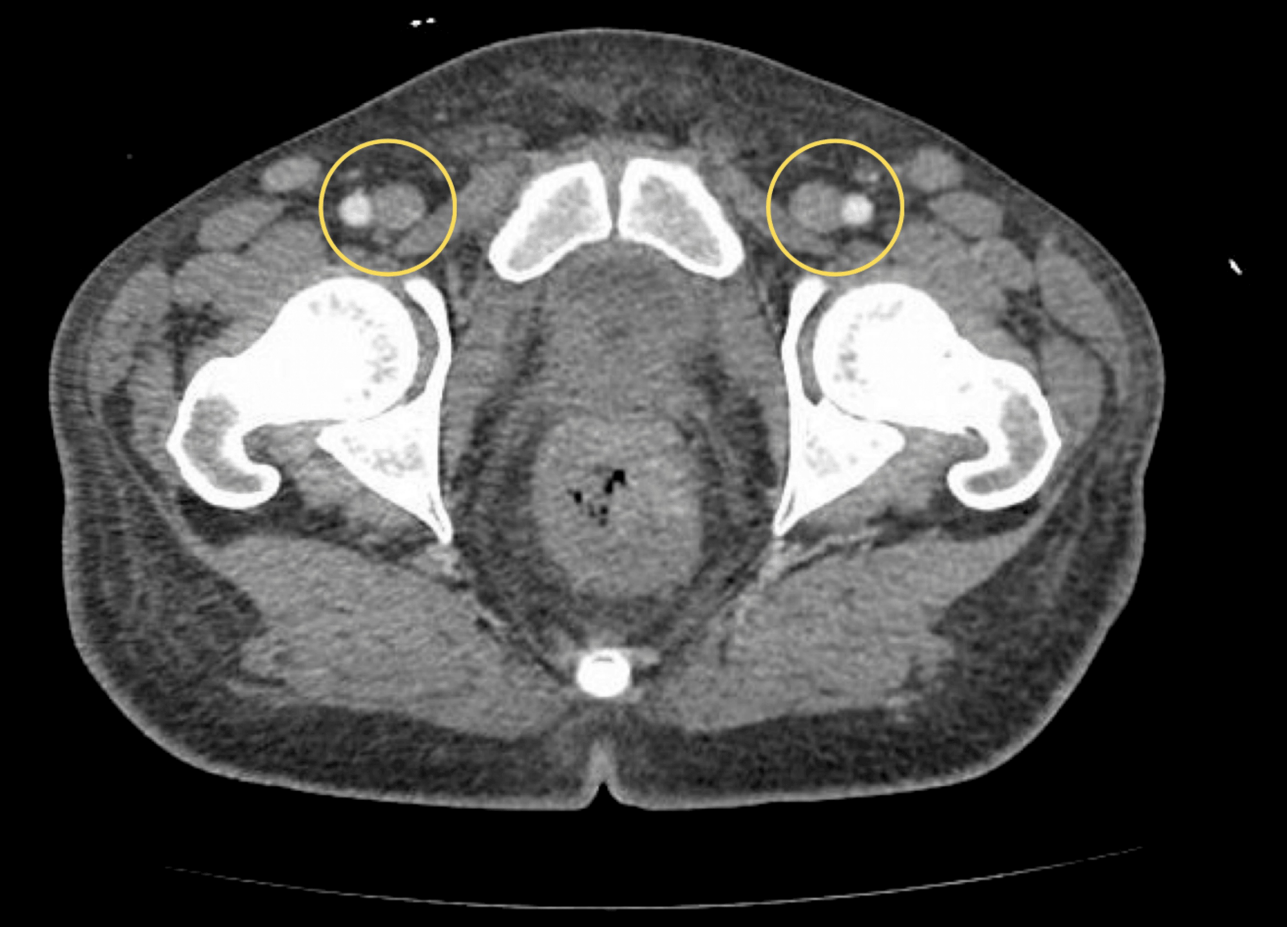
CT scan demonstrating extensive inguinal lymphadenopathy (yellow circles). CT: Computed tomography
